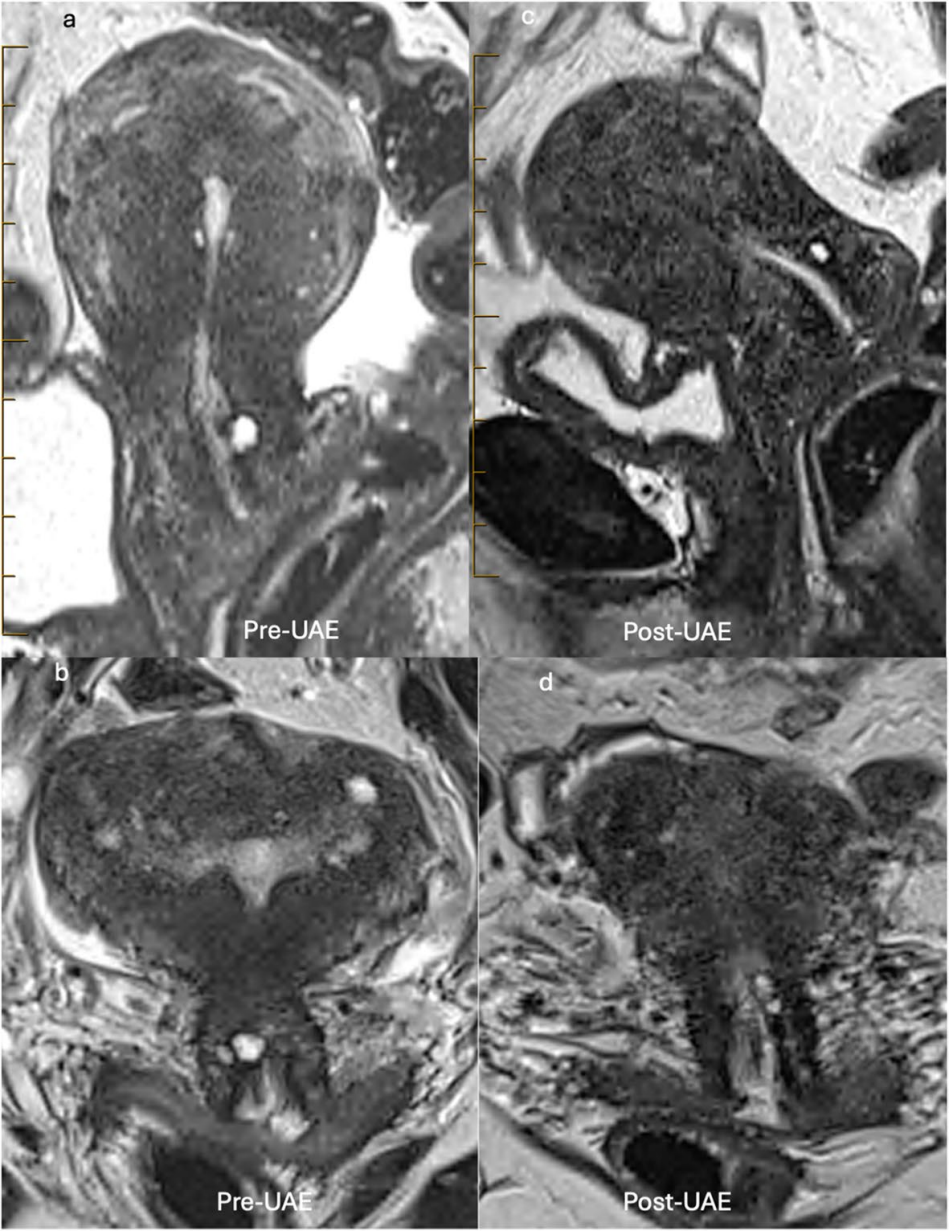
MRI of a 50-year-old woman who had endometrial ablation 10 months ago but was still suffering from heavy menstrual bleeding and severe dysmenorrhea. Following UAE, she had regular periods with lightest-ever bleeding and a reduction of pain score from 10 to 1. Sagittal T2 MRI pre- and post-embolisation demonstrated a reduction of uterine volume from 210 to 97 mL, reduction of junctional zone from 21 to 14 mm, less bulging globular appearance, and marked reduction of myometrial cystic spaces (ectopic endometrial tissue)
